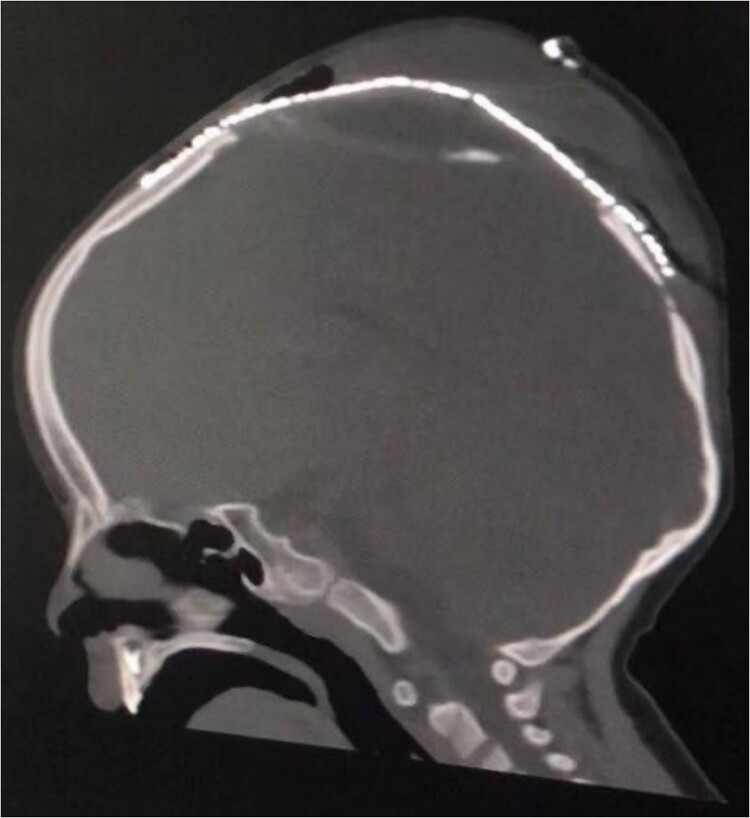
Postoperative sagittal brain CT demonstrating that the brain was inside the cranium and covered by the cranial mesh, preventing further bulging.
